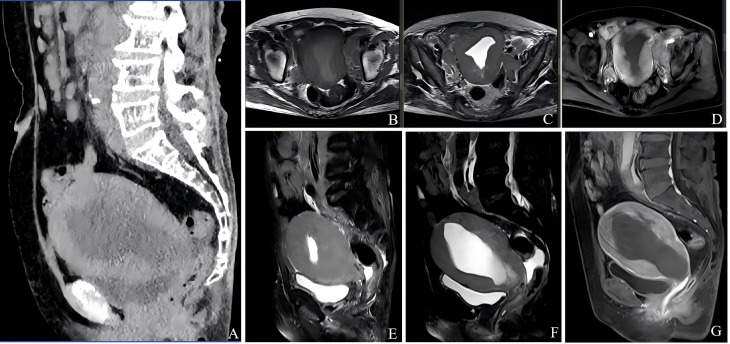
Imaging findings of uterine diffuse large B-cell lymphoma. (A) Contrast-enhanced abdominal CT reveals an enlarged uterus containing a large fluid collection (approximately 4.9 cm × 11.2 cm in the sagittal plane; attenuation ~15 HU). Multiple enlarged lymph nodes are present in the mesenteric root, para-aortic region, and bilateral iliac areas. (B–G) Pelvic MRI demonstrates uterine enlargement with inhomogeneous myometrial thickening and intrauterine fluid. (B) Axial T1-weighted image (T1WI) shows hypointense signal. (C, E, F) T2-weighted images (T2WI) (C) axial; (E, F) sagittal show slightly hypointense signal with patchy areas of T2WI hyperintensity. (D, G) Post-contrast images (D) axial; (G) sagittal demonstrate heterogeneous enhancement of the uterine wall.
